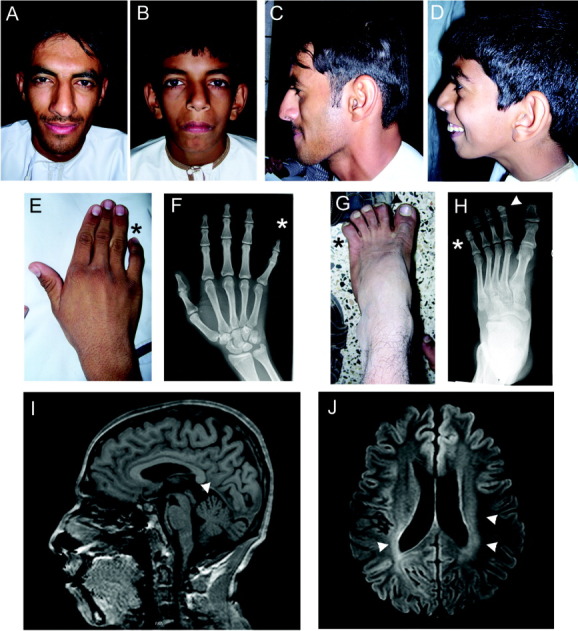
Morphological and radiological features of Omani individuals with Troyer syndrome. Comparison between (A, C) an unaffected and (B, D) an affected individual revealed (A, B) relative hypertelorism and (C, D) pronounced overbite. Examples of skeletal anomalies in the extremities: brachydactyly (short digits; E, F), camptodactyly (flexed digit; *asterisks* in E, F), hammer toes (*asterisks* in G, H), and clinodactyly (curved digit; *arrowheads* in G, H). Brain magnetic resonance imaging shows atrophy of the cerebellar vermis (*arrowhead* in I) and white matter hyperintensity in T2-weighted images, which is more prominent posteriorly (*arrowheads* in J).
